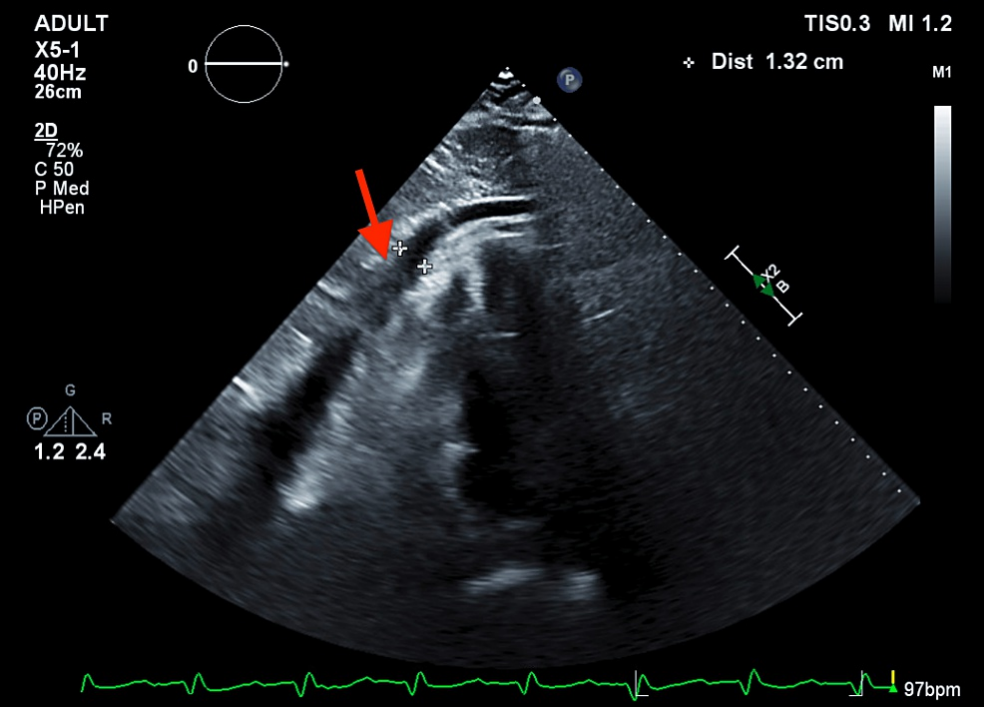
Echocardiogram four-chamber view with the red arrow pointing toward coagulum in pericardial effusion.
